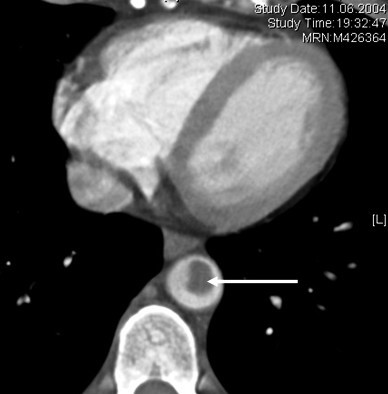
Thoraco-abdominal CT-scan image showing the thrombus in the thoracic aorta (arrow).
